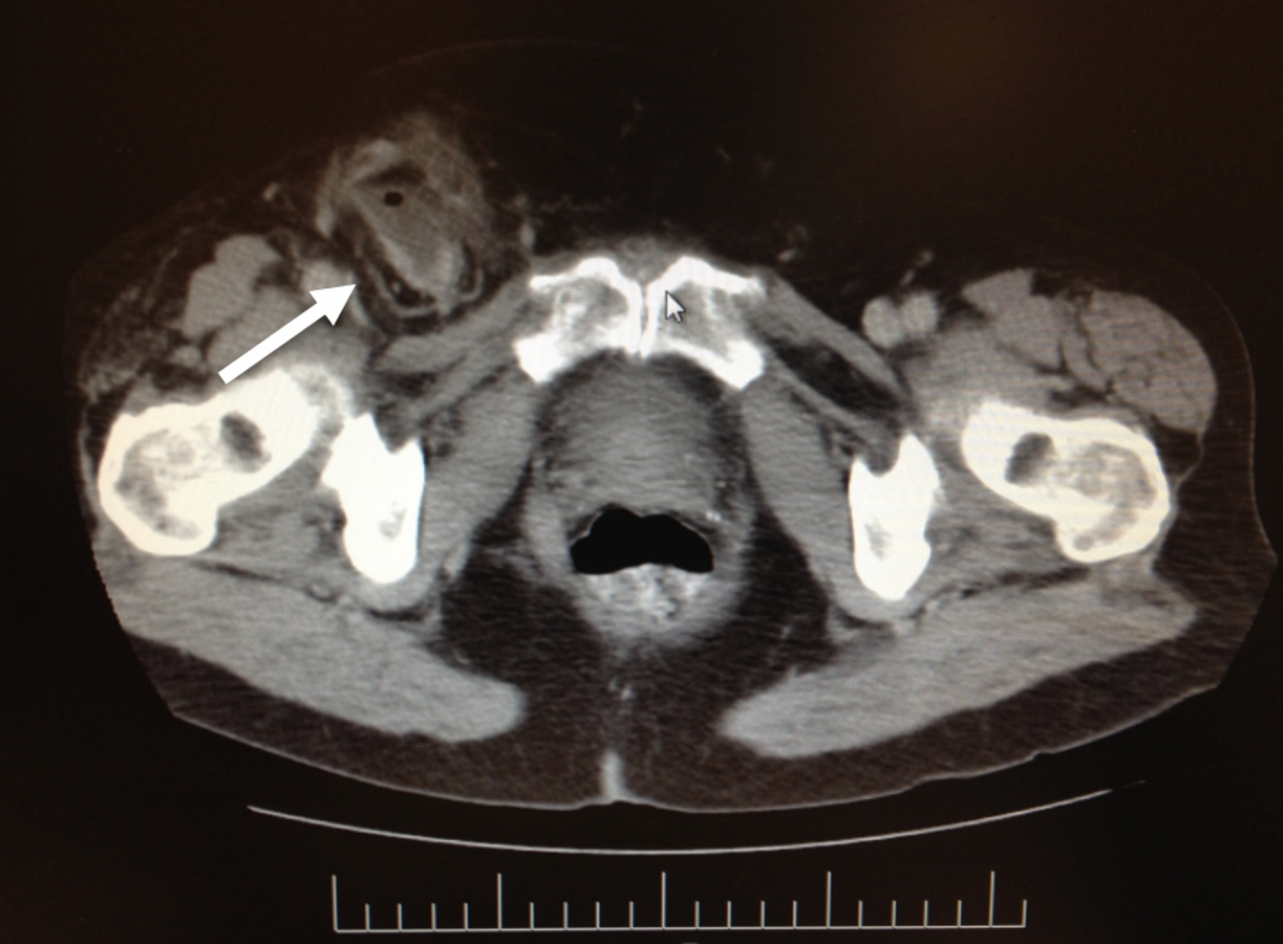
A CT scan demostrating the appendix herniating into right inguinal hernia with inflammatory changes surrounding it CT = computed tomography
